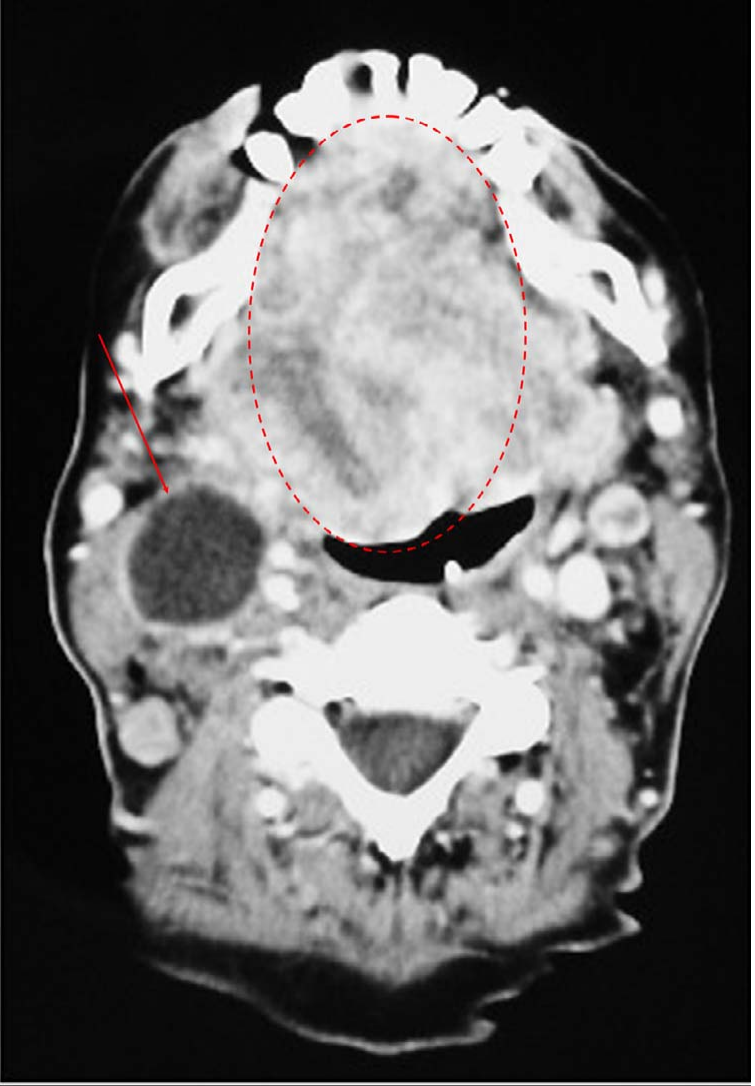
Contrast enhanced CT, soft tissue window: Tumor of the tongue (10 cm) with a large lymphe node metastasis near the right cervical vessel-nerve-sheath (red arrow).
